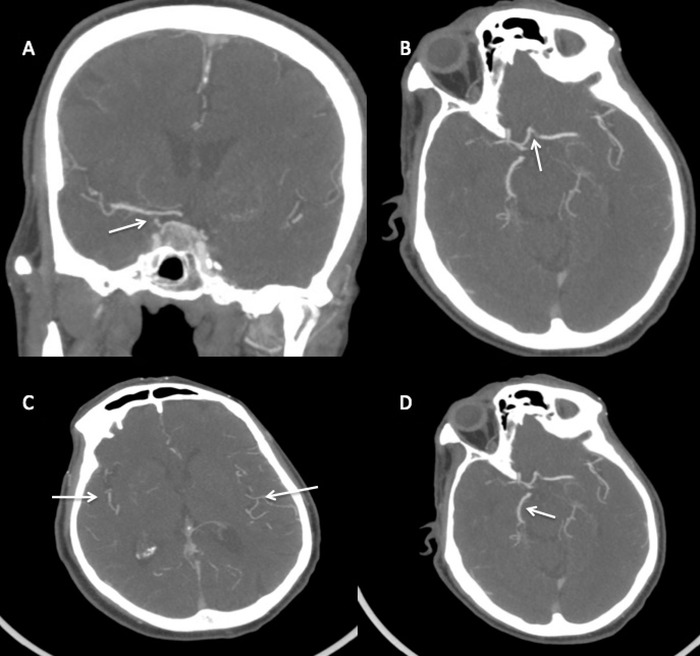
(A) Coronal computed tomography (CT) angiography of the head demonstrates short segment occlusion of the right supraclinoid segment of the internal carotid artery (arrow). (B) Axial view shows collateral flow from contralateral anterior circulation across the anterior communicating artery (arrow). (C) The bilateral middle cerebral arteries fill completely on axial CT angiography without branch occlusions (arrows), as does (D) the right posterior cerebral artery through the anterior communicating artery (arrow).
